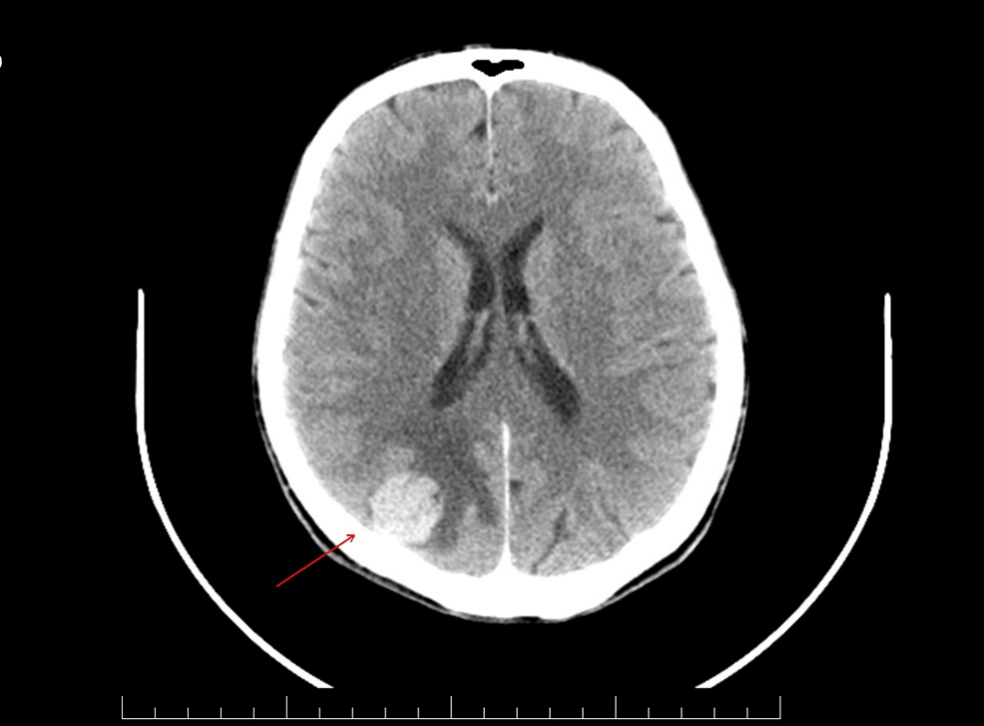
Cerebral MRI axial view. In the occipital area of the right main hemisphere, a suspicious lesion with a signal can be visualized on magnetic resonance imaging. It appears moderately increased on T2 and fluid-attenuated inversion recovery (FLAIR) sequences, isotensive on T1, and narrow on diffusion-weighted imaging (DWI), measuring 27 mm anteroposterior diameter, 22 mm laterolateral diameter, and 23 mm craniocaudal diameter. Additionally, a pathological contrast accumulation area of 2 mm in diameter is observed in the cortico-subcortical area of the main cerebral hemisphere, suggesting the appearance of brain metastasis.
